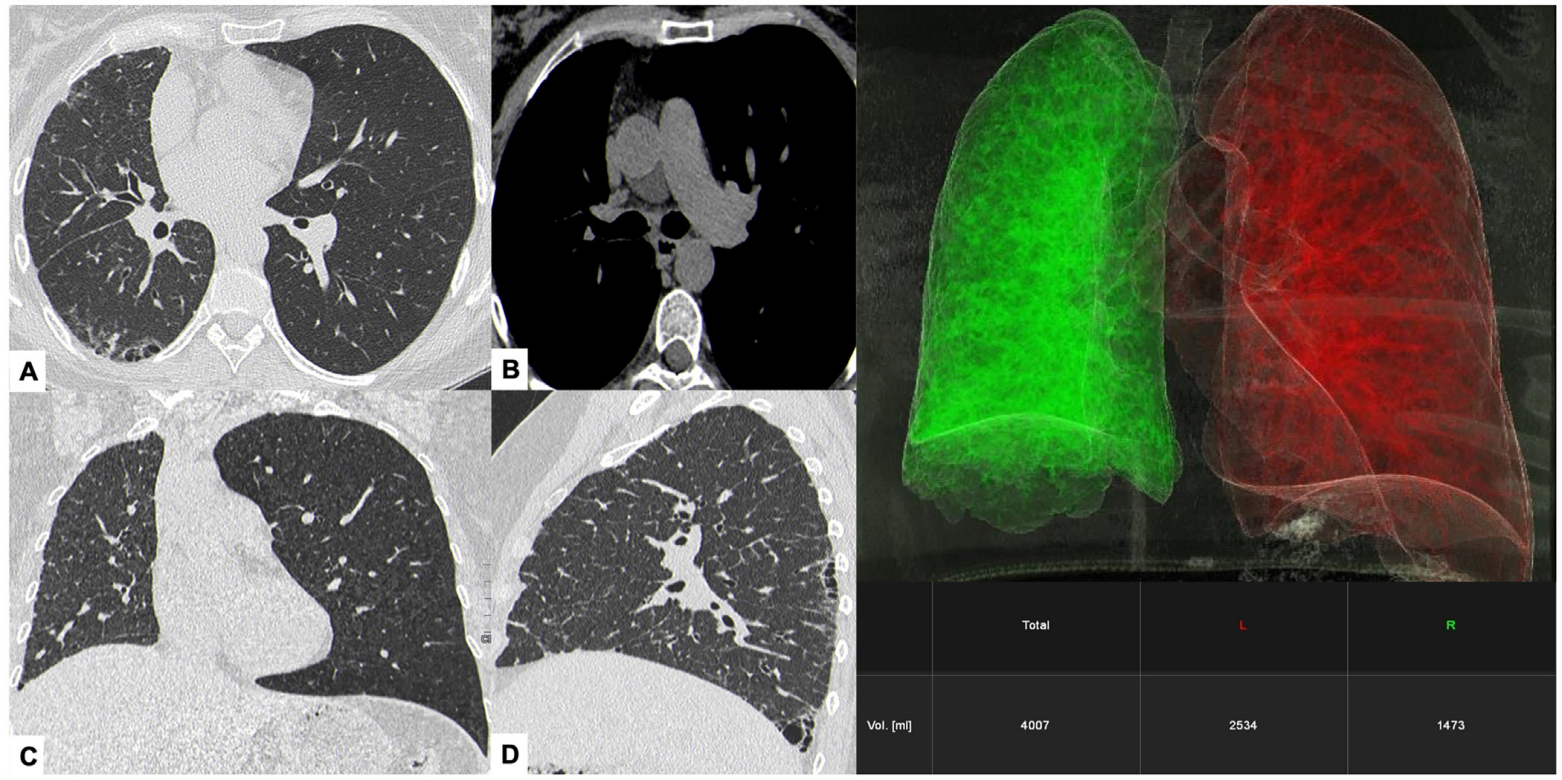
Case 1 – Unilateral absence of the right pulmonary artery (UAPA) causing unilateral pulmonary fibrosis.* HRCT images show irregular subpleural interstitial thickening and honeycombing-like foci confined to the right lung (white arrows in A, B), resulting in marked asymmetry of lung inflation (C). Mediastinal window reconstruction demonstrates complete agenesis of the right pulmonary artery (arrowhead in D), identified as the underlying cause of the unilateral fibrotic changes. The 3D volumetric reconstruction (right panel) generated using Siemens Healthcare software (syngo.via Pulmo3D) confirms reduced right lung volume compared to the left (1473 mL vs. 2534 mL).
